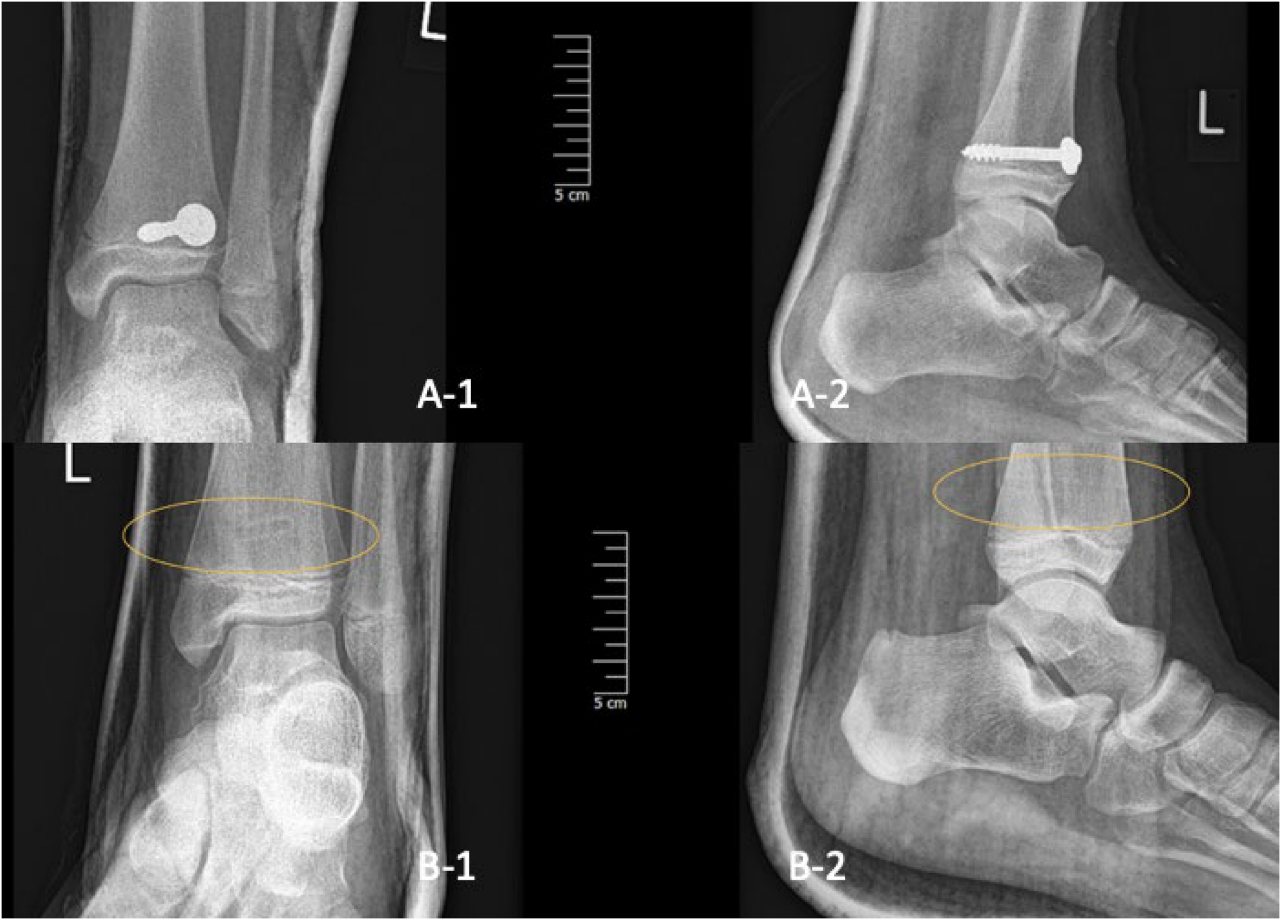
Two-plane radiographs of SH-II fracture one-day post-op in one child treated with metal (A-1/2) and in another treated with PLGA implants (B-1/2), the implant is marked with the yellow oval.
